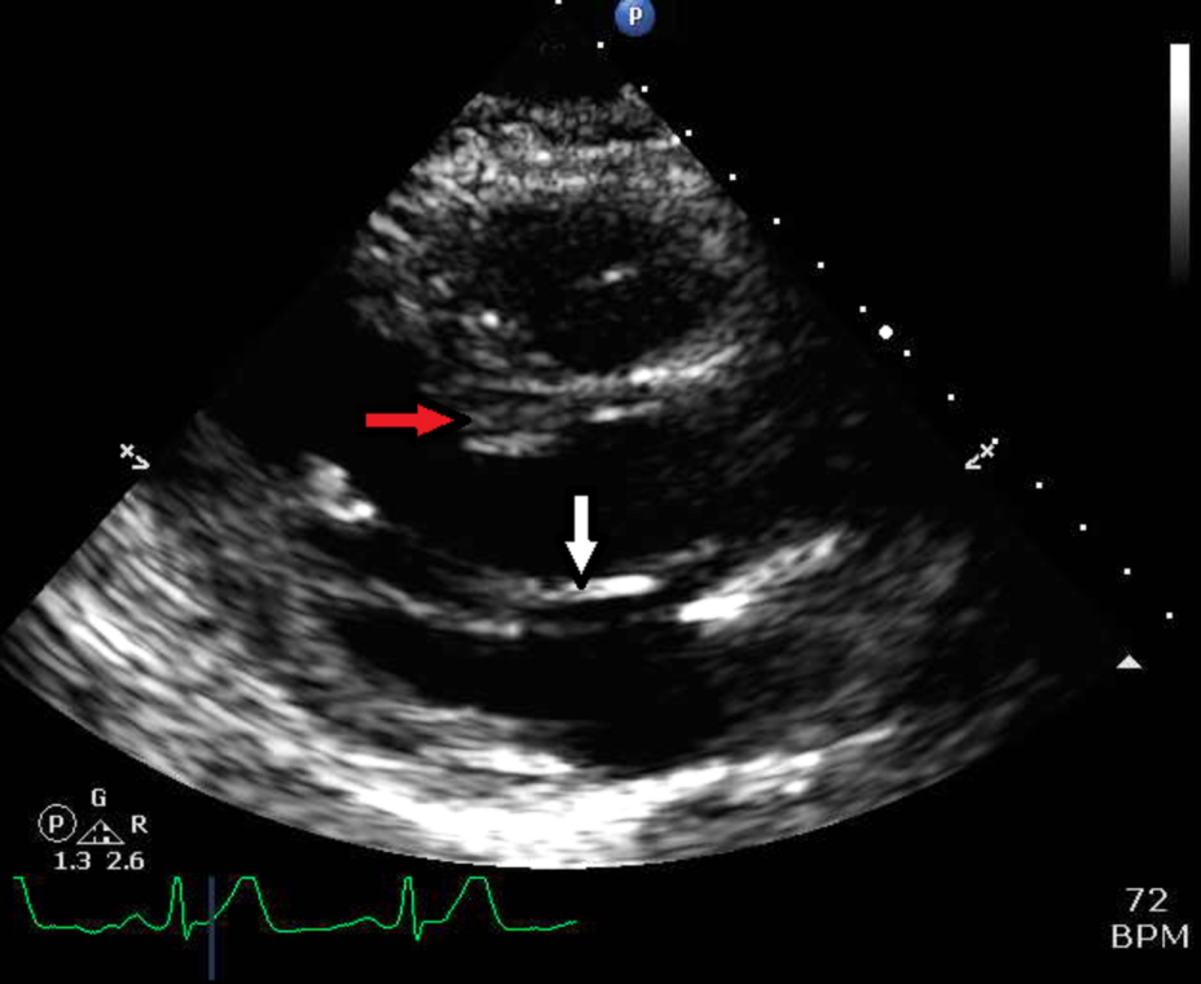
Next-day transthoracic echocardiographic image showing no contact between anterior mitral valve leaflet (white arrow) and the interventricular septum (red arrow)
